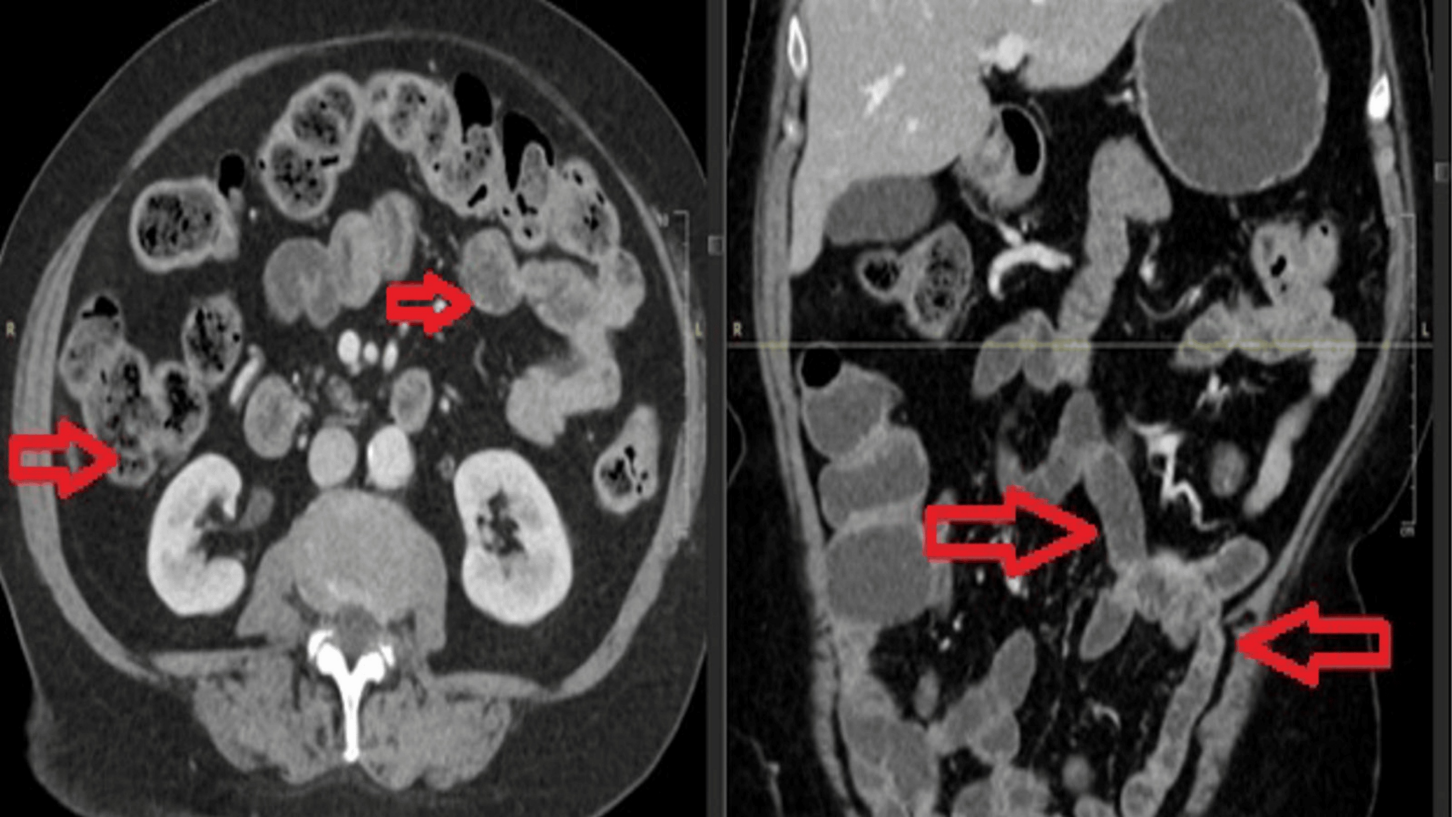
Contrast-enhanced CT images of the abdomen in axial (left) and coronal (right) views demonstrate matted loops of small bowel with wall thickening and increased mucosal enhancement (indicated by arrows). These findings are consistent with chronic enteritis. In the context of an immunocompromised patient with persistent diarrhea, such features raise a strong suspicion for viral enteritis, including sapovirus infection. CT: computed tomography
